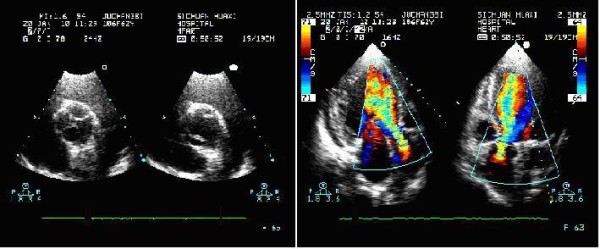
The preoperative transthoracic echocardiogram (TTE) disclosed a moderate to severe aortic valve insufficiency but failed to reveal the quadricuspid aortic valve anomaly clearly.
